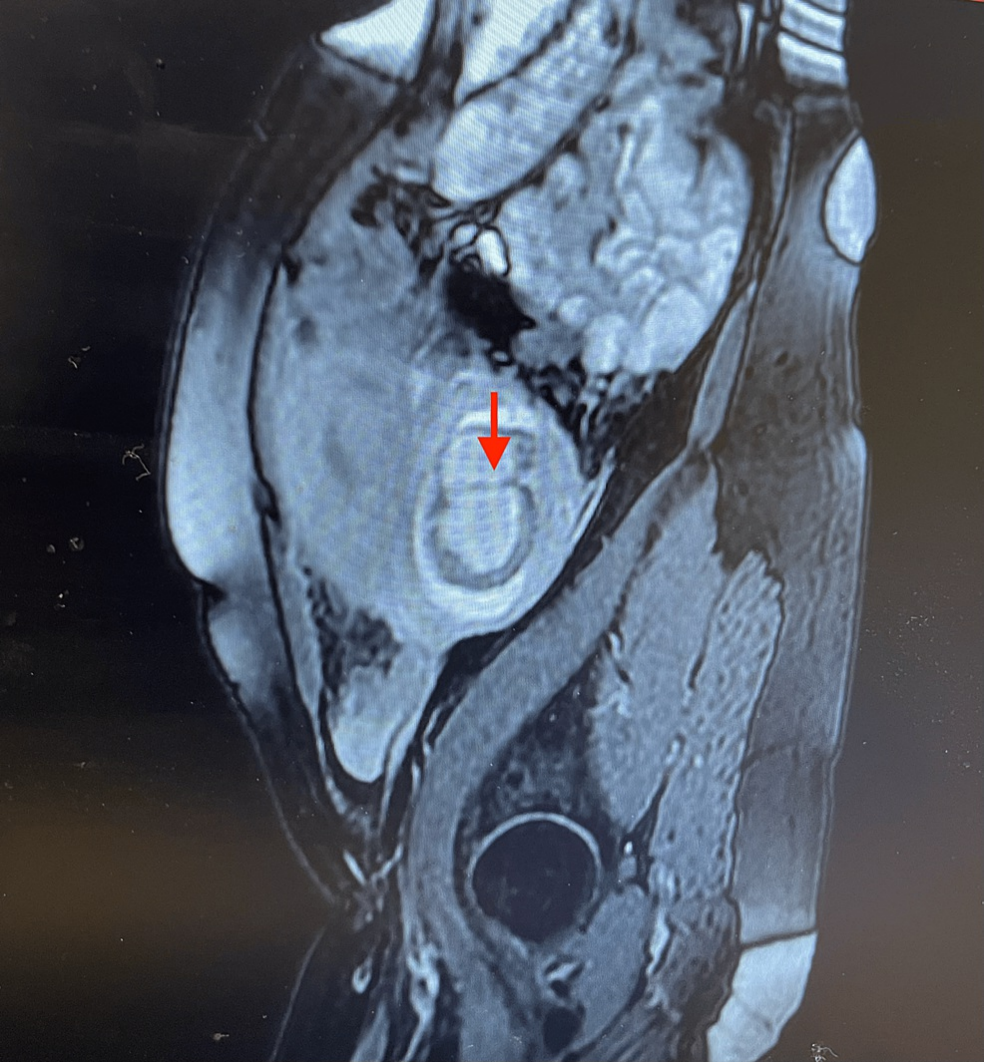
A sagittal-plane MRI of the abdomen and pelvis. The image describes sagittal plane magnetic resonance imaging (MRI) showing a complex cystic left adnexal mass adhering to the uterus (marked with a red arrow).
